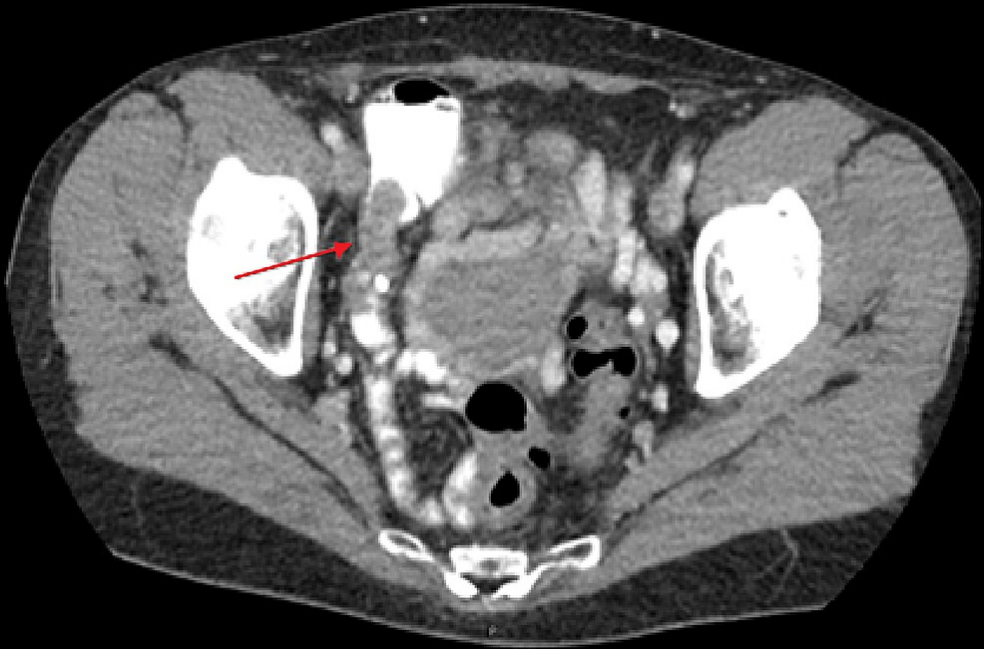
Staging CT scan prior to surgical intervention shows no signs of metastatic disease. Red arrow shows mass in the appendix extending into the colon.
